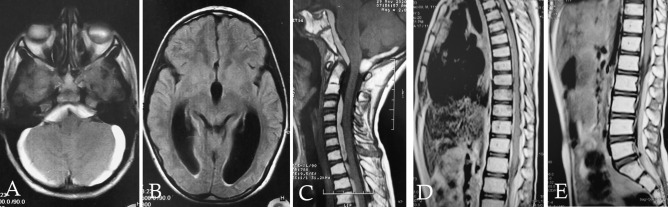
Initial MRI of the whole craniospinal axis at the time of presentation. A Posterior fossa T1W axial image showing posterior fossa and peri-mesencephalic SDH, B supratentorial T1W axial image showing supratentorial obstructive hydrocephalus, C T1W sagittal image showing SDH extending anteriorly opposite the whole clivus and posteriorly opposite the surface of both cerebellar hemispheres and extending anteriorly to the whole cervical spinal cord, D thoracic spine T1W sagittal image showing SDH extending anteriorly to the thoracic spinal cord, and E lumbosacral T1W sagittal image showing SDH extending posteriorly to the cauda equina down to the level of the lower end of the sacrum and associated with cauda equina compression
